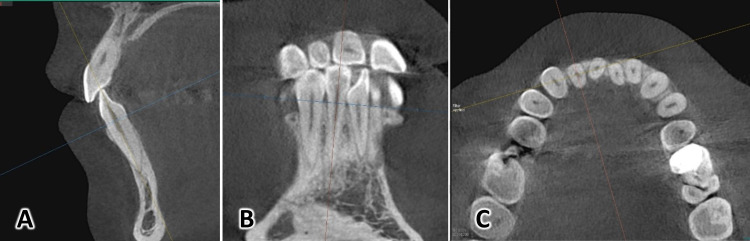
Orienting the CBCT images for the detection and measurement of fenestrations and dehiscences First, the axial plane (in blue) was moved to pass through the CEJ from the buccal and lingual sides of each tooth of the lower anterior teeth as can be seen in the sagittal (A) and coronal views (B).  Then, the coronal plane (in orange) was moved until it became parallel to the vertical axis of each examined tooth passing from the incisal edge to the apex of the root (A). Afterwards, the sagittal plane (in red) was adjusted until it passed the middle of the incisal edge and the root apex (B and C).
